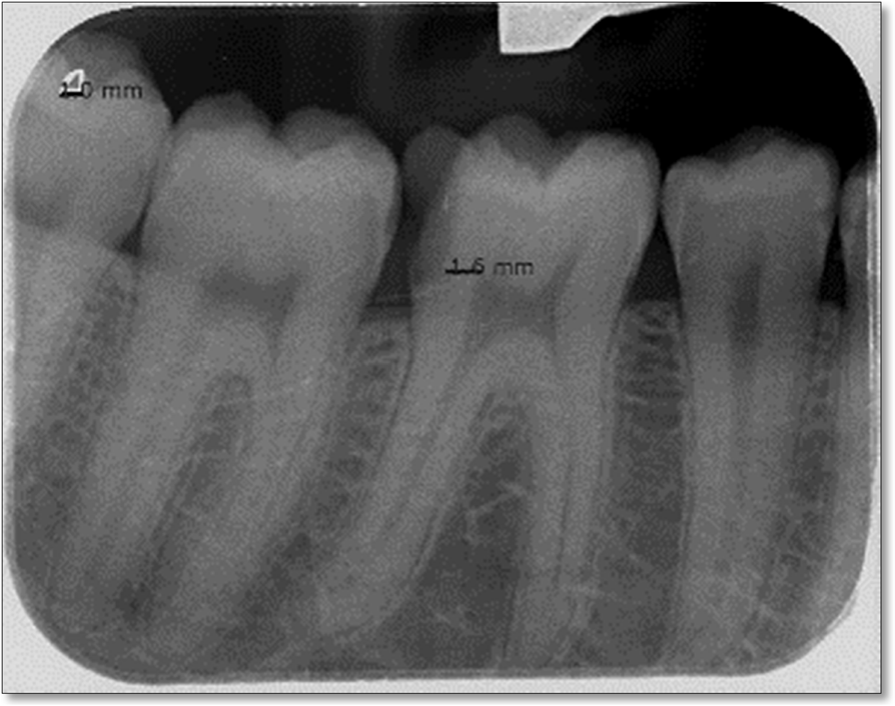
A radiograph showing the measurement of the RDT (1.6 mm) on the distal of the lower right first molar. Note the triangle in the upper border of the radiograph was used as a reference
